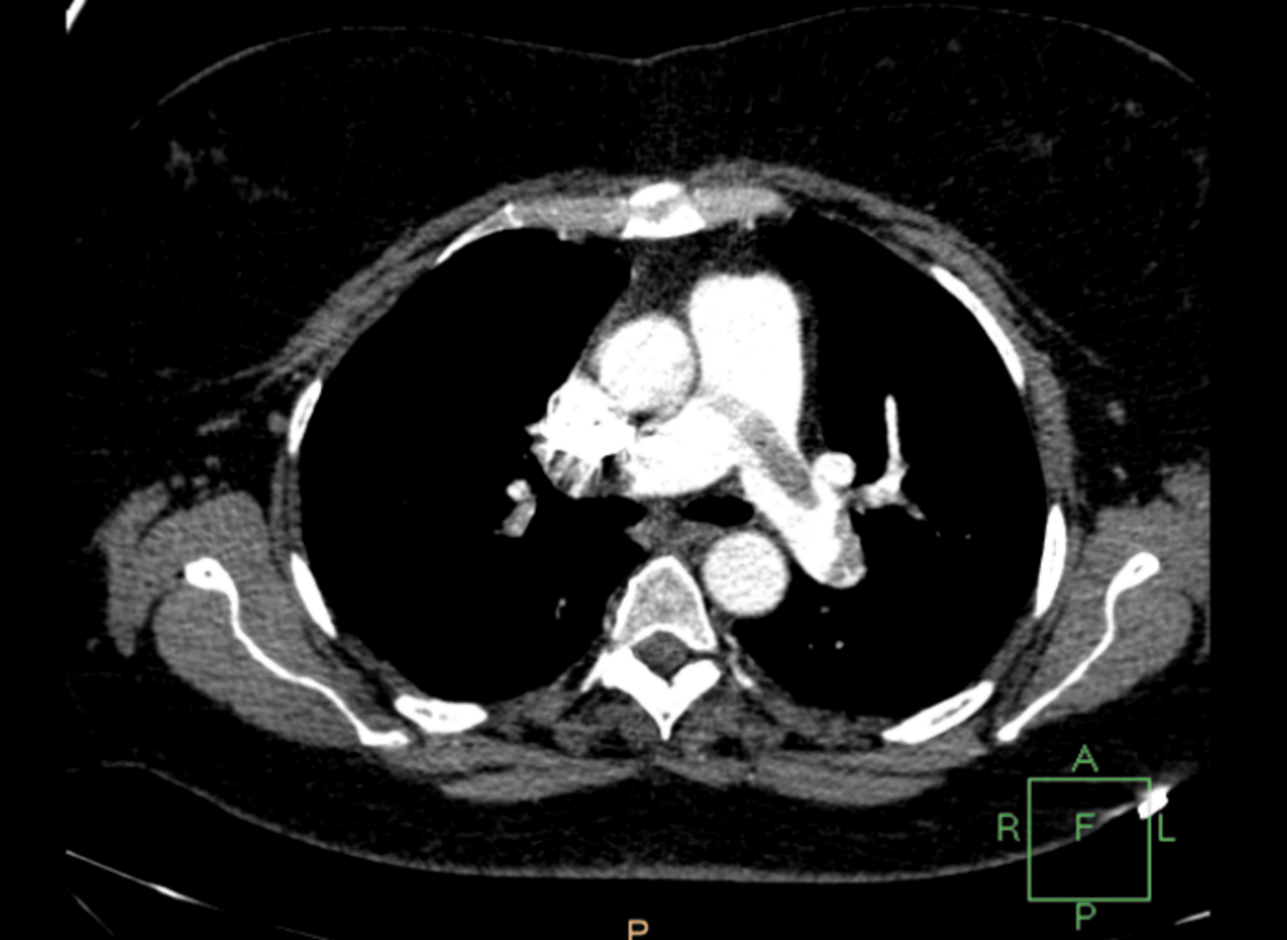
Computed tomography angiography showing pulmonary embolism
